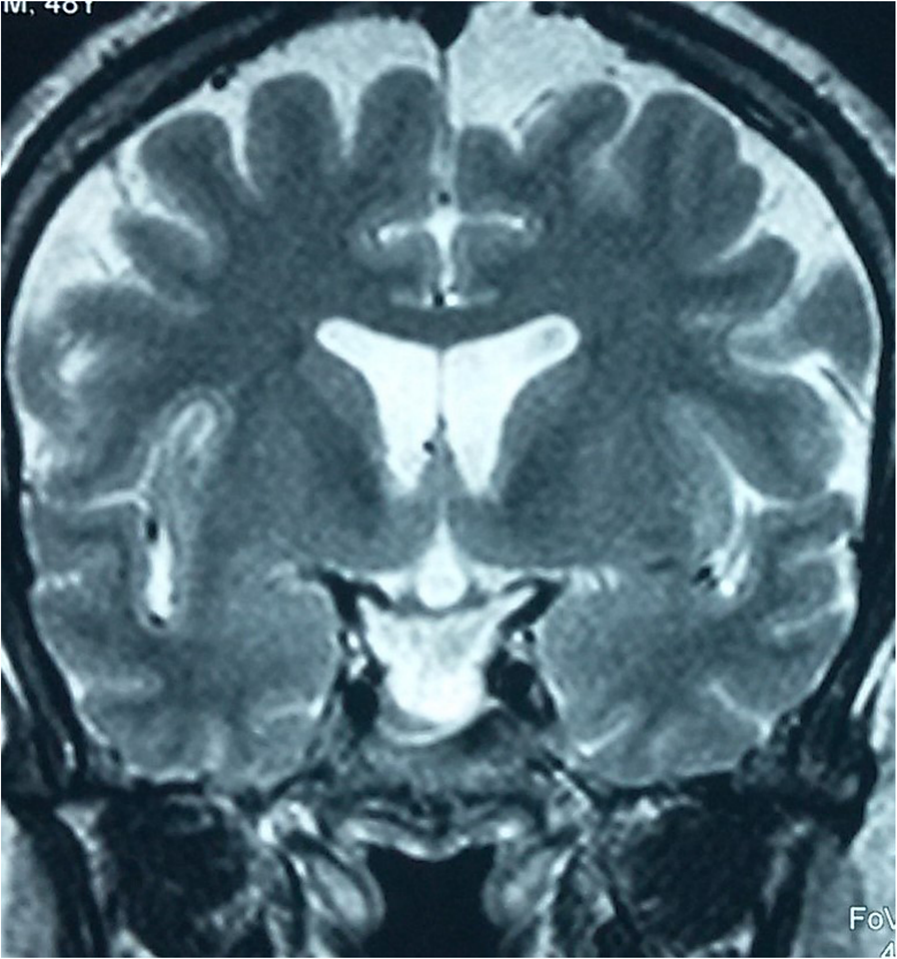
T2-weighted magnetic resonance imaging scan (coronal cut) showing the empty sella
